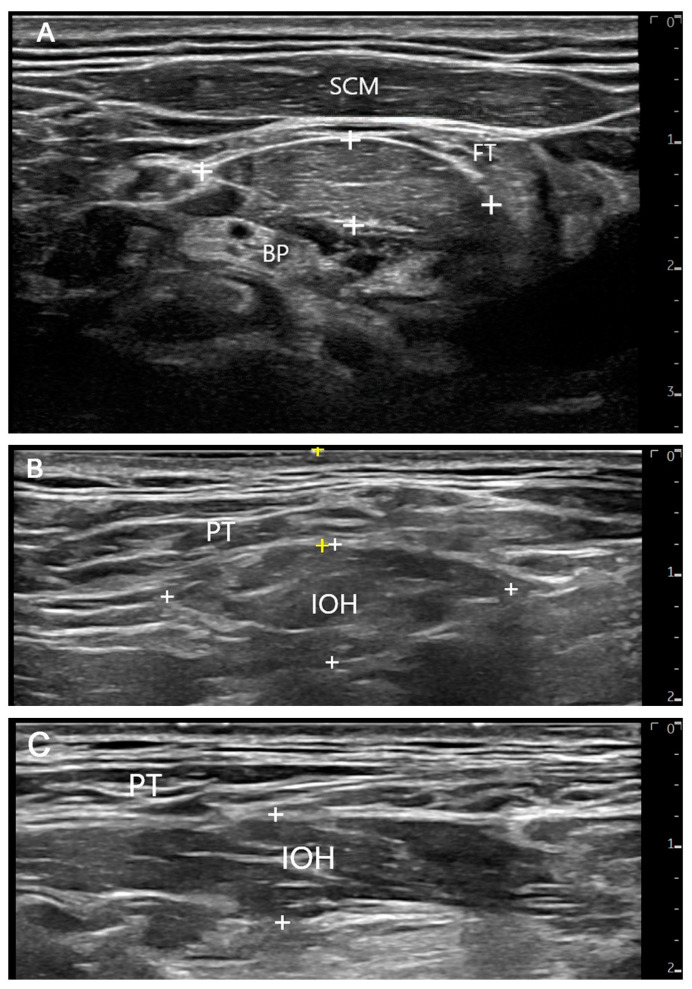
Ultrasound of inferior belly of omohyoid. (A) The inferior belly of omohyoid (IOH) between crosses in short axis (transverse), close to the intermediate tendon of omohyoid and beneath sternocleidomastoid (SCM) (FT, fat tissue; BP, brachial plexus). (B) Short-axis scan of the IOH (between white crosses) and distance between the IOH and the skin (between yellow crosses) at mid-clavicle. (C) Long-axis (longitudinal) scan of the IOH (between white crosses). PT, platysma. The scale bar indicating centimeters is shown on the right side of the ultrasound images.
